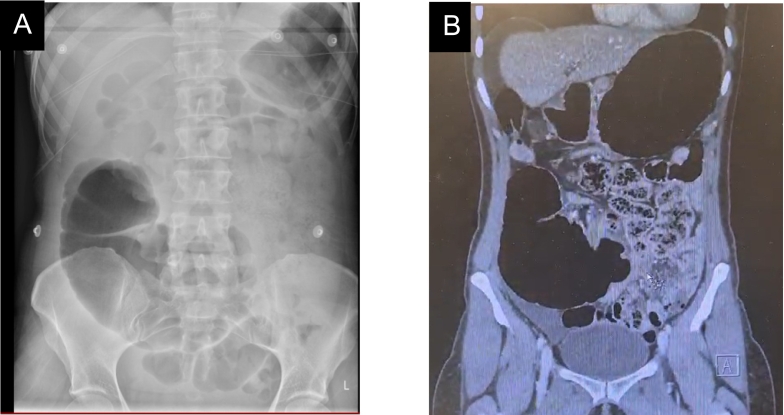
Abdominal Xray and CT abdomen pelvis of Case 2.
